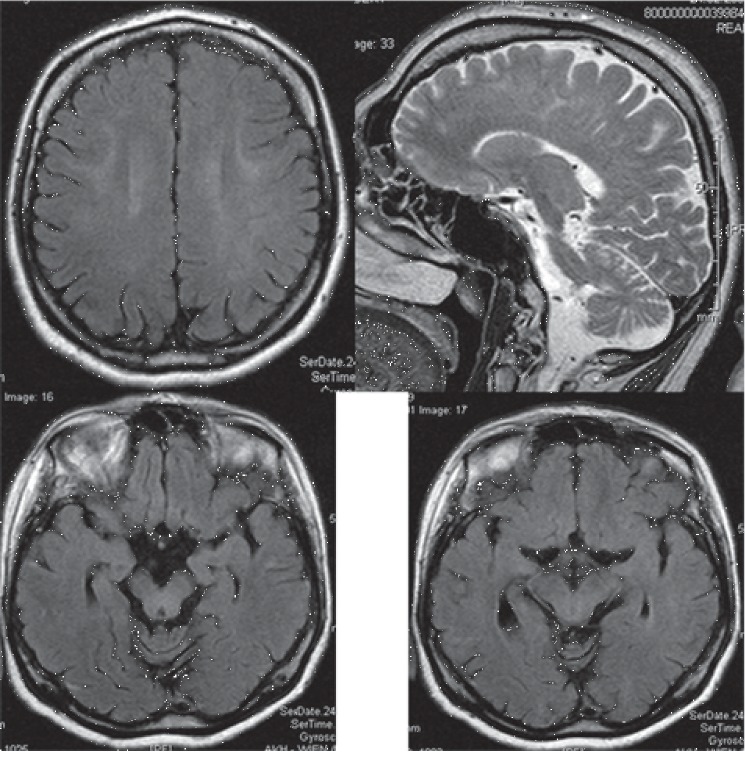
Cerebral MRI at age 36 showing mild, band-like T2-hyperintensities in the white matter bilaterally (upper left) and the midbrain (lower panels) and mild cerebellar atrophy (upper right).
